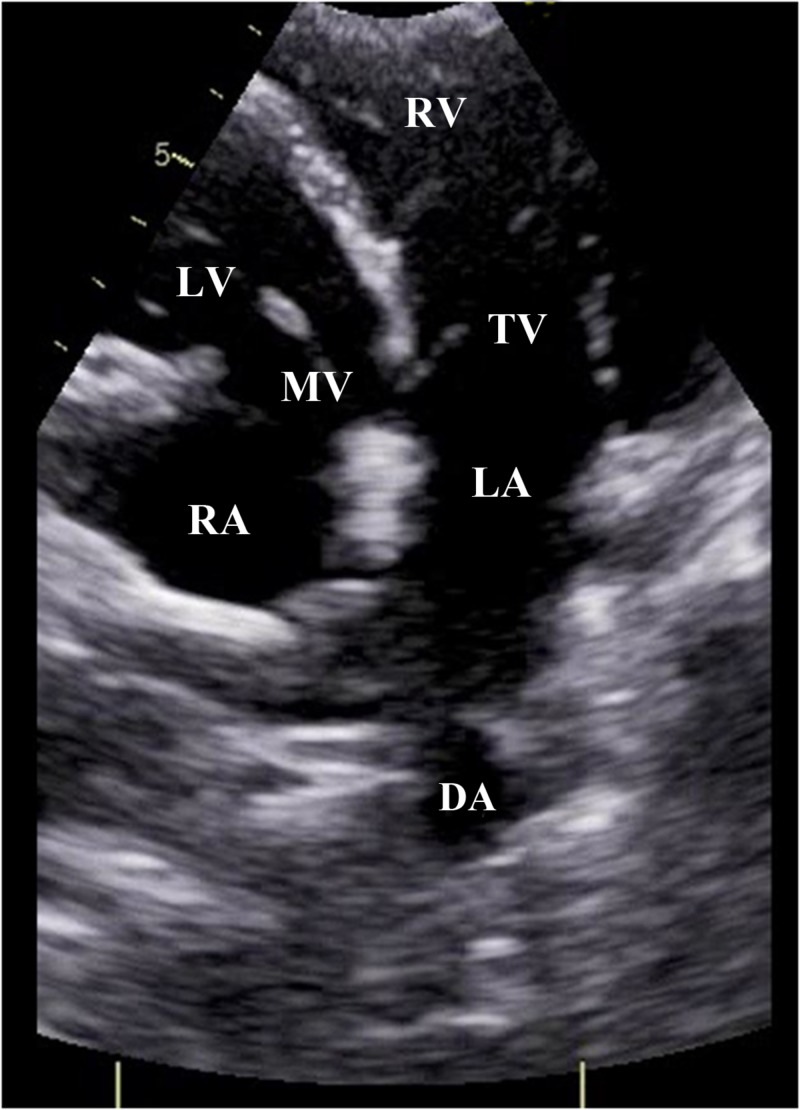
Echocardiogram. Apical four chamber view showing right atrium (RA) connected to left ventricle (LV) through mitral valve (MV). Left atrium (LA) is connected to right ventricle (RV) through tricuspid valve (TV). Descending aorta (DA) is also seen.
